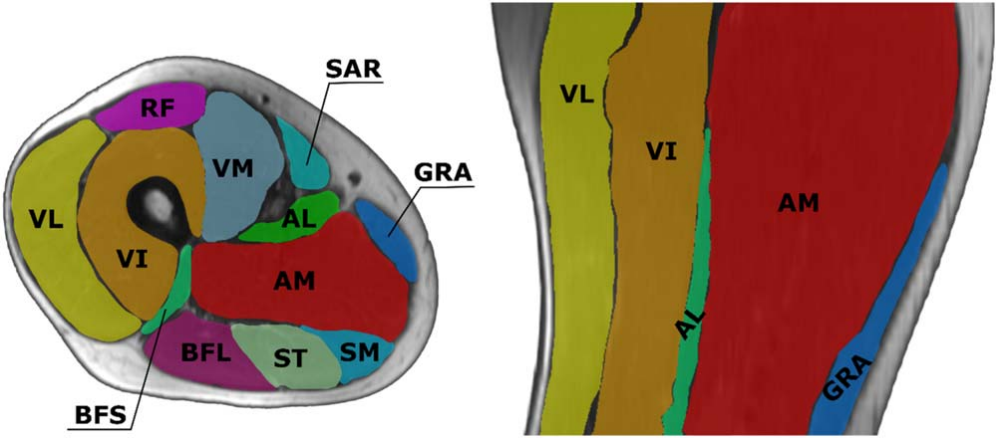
Representative T1-weighted MR anatomical image of the thigh, axial and sagittal views. The 12 muscles are: sartorius SAR, vastus medialis VM, vastus intermedius VI, vastus lateralis VL, rectus femoris RF, biceps femoris short head BFS, biceps femoris long head BFL, semitendinosus ST, gracilis GRA, semimembranosus SM, adductor longus AL, adductor magnus AM.
